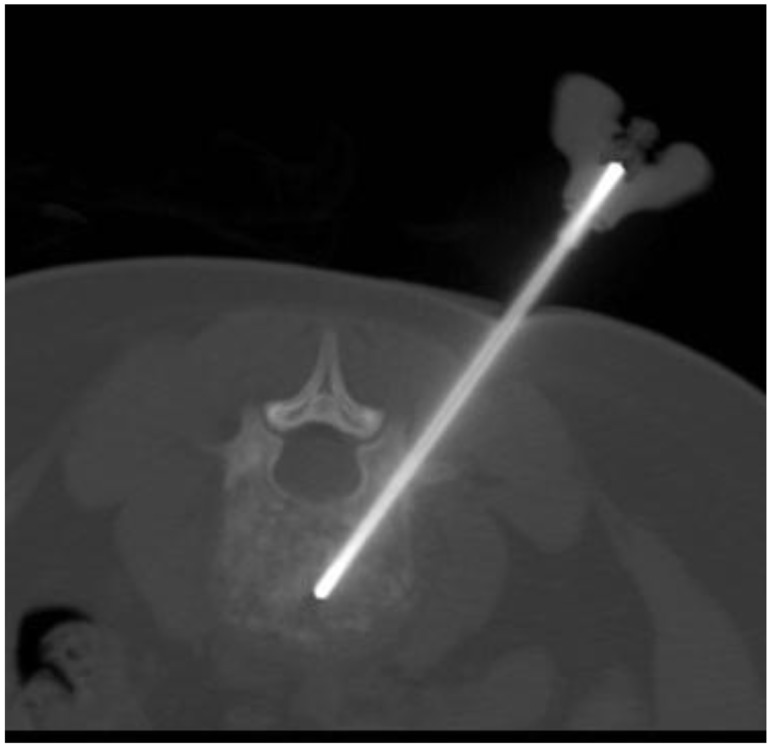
Interventional biopsy of a bone lesion: this figure depicts a CT image of a vertebral bone biopsy using an 11-gage biopsy needle. Optimum tumor acquisition is feasible with a smaller number of passes.
